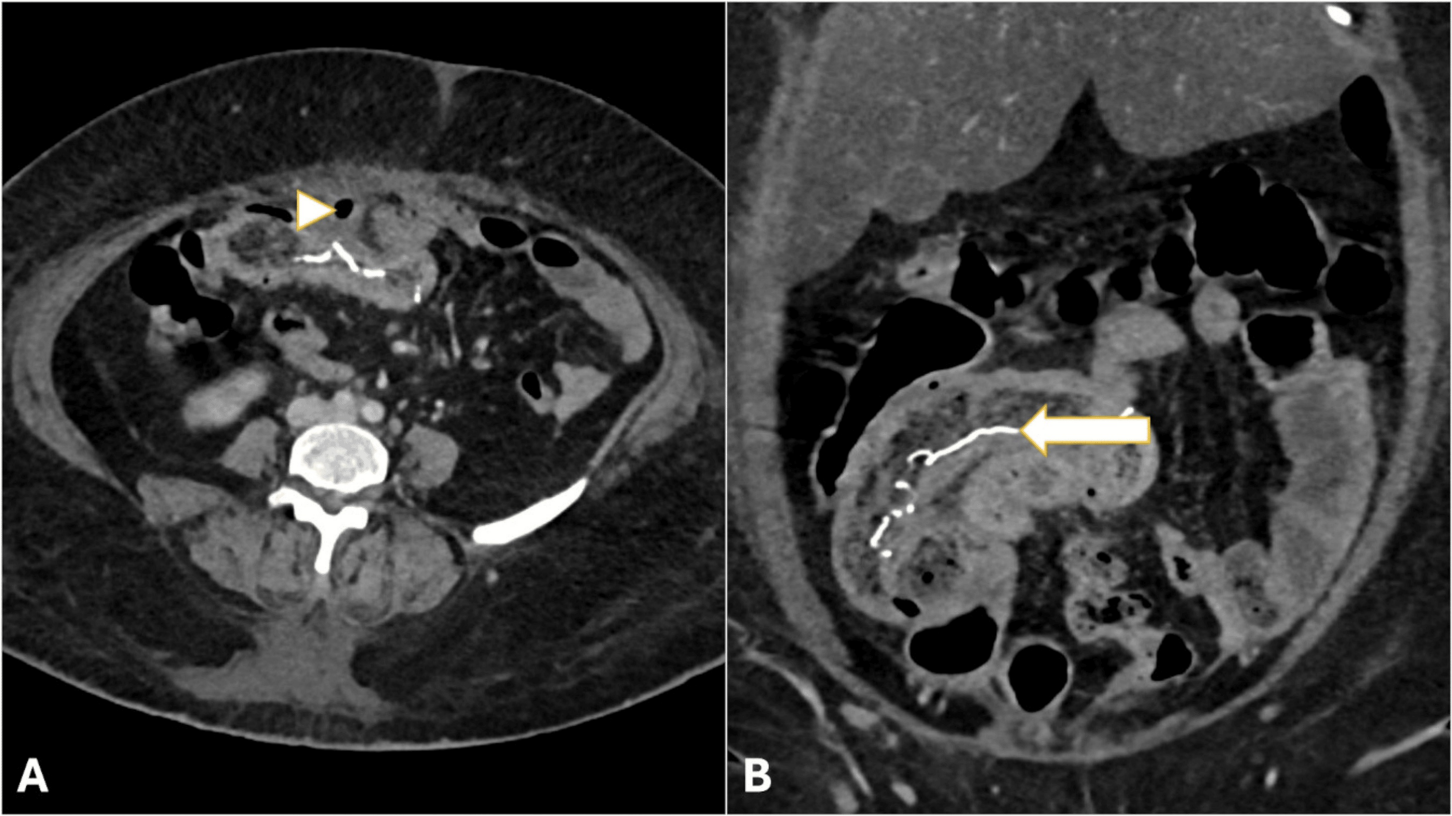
Contrast-enhanced CT images showing a circumferential mural thickening of the distal ileal loop with internal mottled gas lucencies. (A) Axial image: adjacent extraluminal air foci are indicated by the arrowhead. (B) Coronal reformatted image: a serpiginous hyperdense structure, likely representing the radiopaque marker of the retained surgical sponge, is indicated by the arrow. Surrounding mesenteric fat stranding is also noted, consistent with a contained perforation. CT: computed tomography
